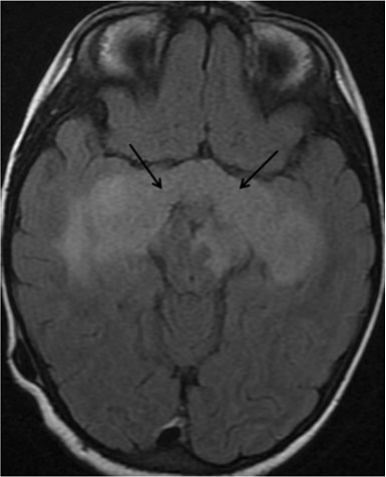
A 3-year-old child with visual difficulties. Axial FLAIR image show a supra-sellar lesion extending to the temporal lobes along the optic tracts (*arrows*) with moderate mass effect, compatible with optic glioma. FLAIR hyperintensity is also noted in the left mesencephalon from additional tumoral involvement
